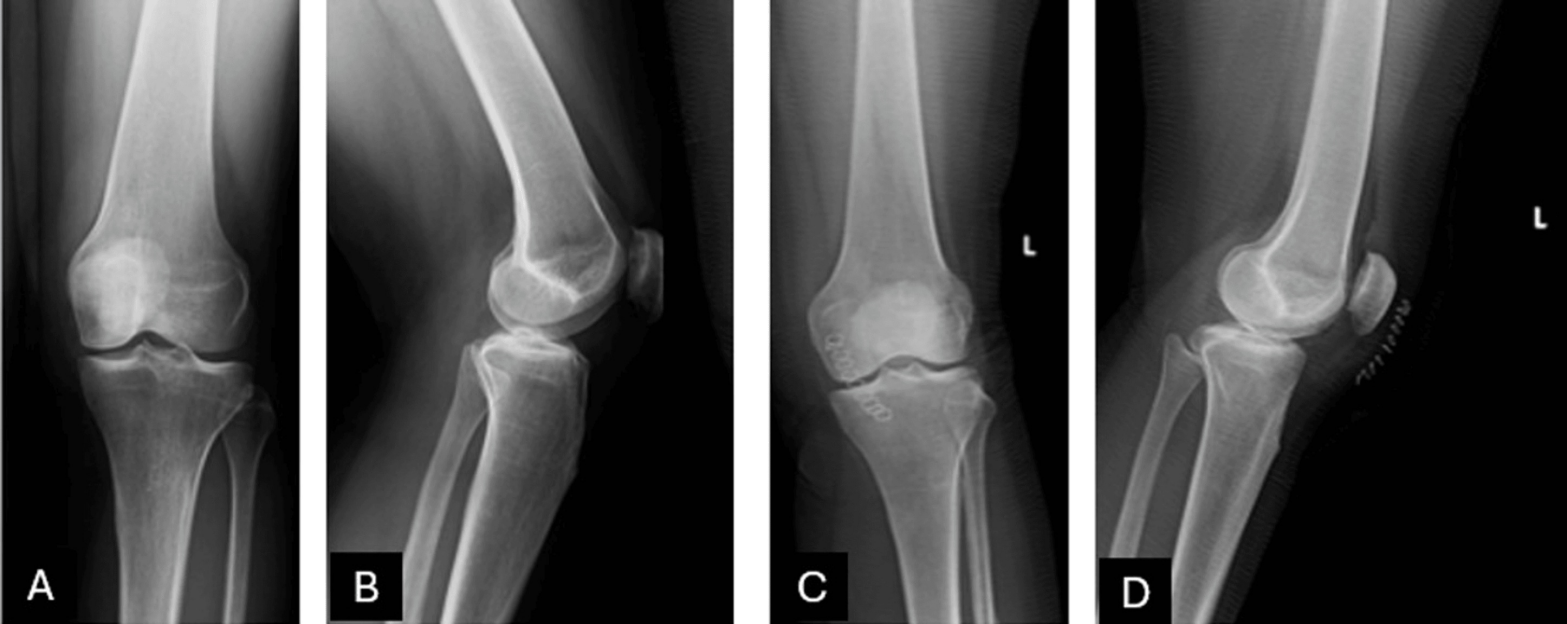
Preoperative and early postoperative radiological images of the patient. (A) Preoperative anteroposterior knee X-ray. (B) Preoperative lateral knee X-ray. (C) Early postoperative anteroposterior knee X-ray. (D) Early postoperative lateral knee X-ray. No significant osseous pathology is observed
